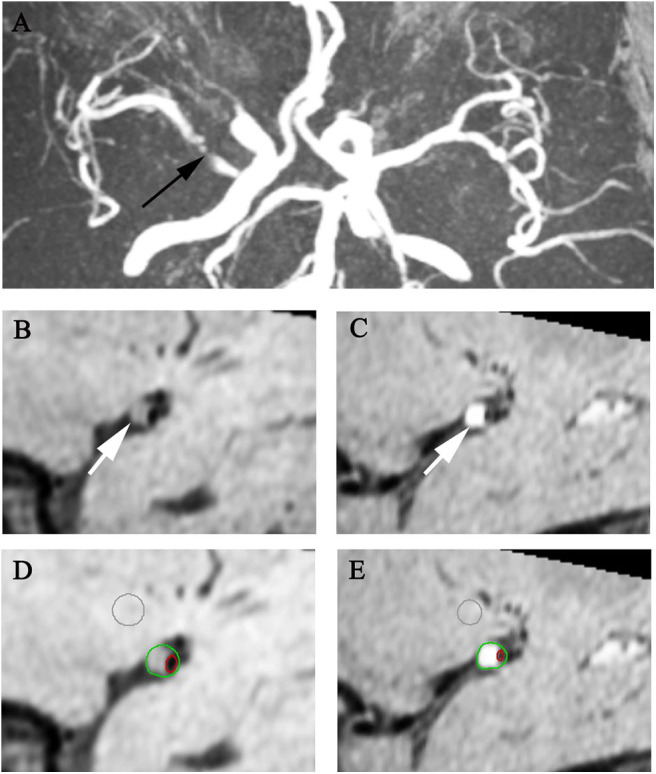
Representative MR images showing enhancement index measurement. A 60-year-old man with acute stroke showed severe right MCA stenosis on MRA (A arrow). After matching pre- (B,D) and post-contrast T1w images (C,E) at the most stenotic site, the plaque enhancement index of the plaque (arrows) is 48.74%.
